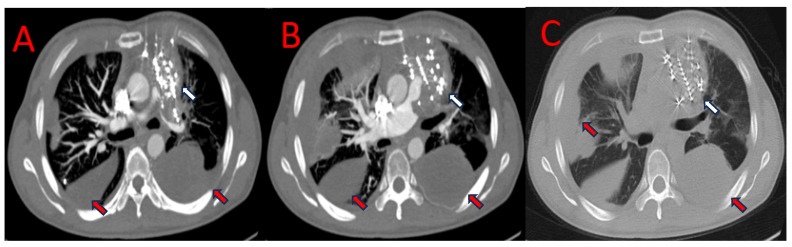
The fifth chest CT scan of the patient in West China Hospital, Sichuan University (6 February 2023). MIP (A,B) and axial CT (C) showed that after radioactive particle implants had been performed (white arrow) the volume of soft tissue masses in the pulmonary artery and anterior mediastinum decreased, but bilateral lung and pleural metastases increased significantly (red arrow). CT, computed tomography; MIP, maximum intensity projection.
